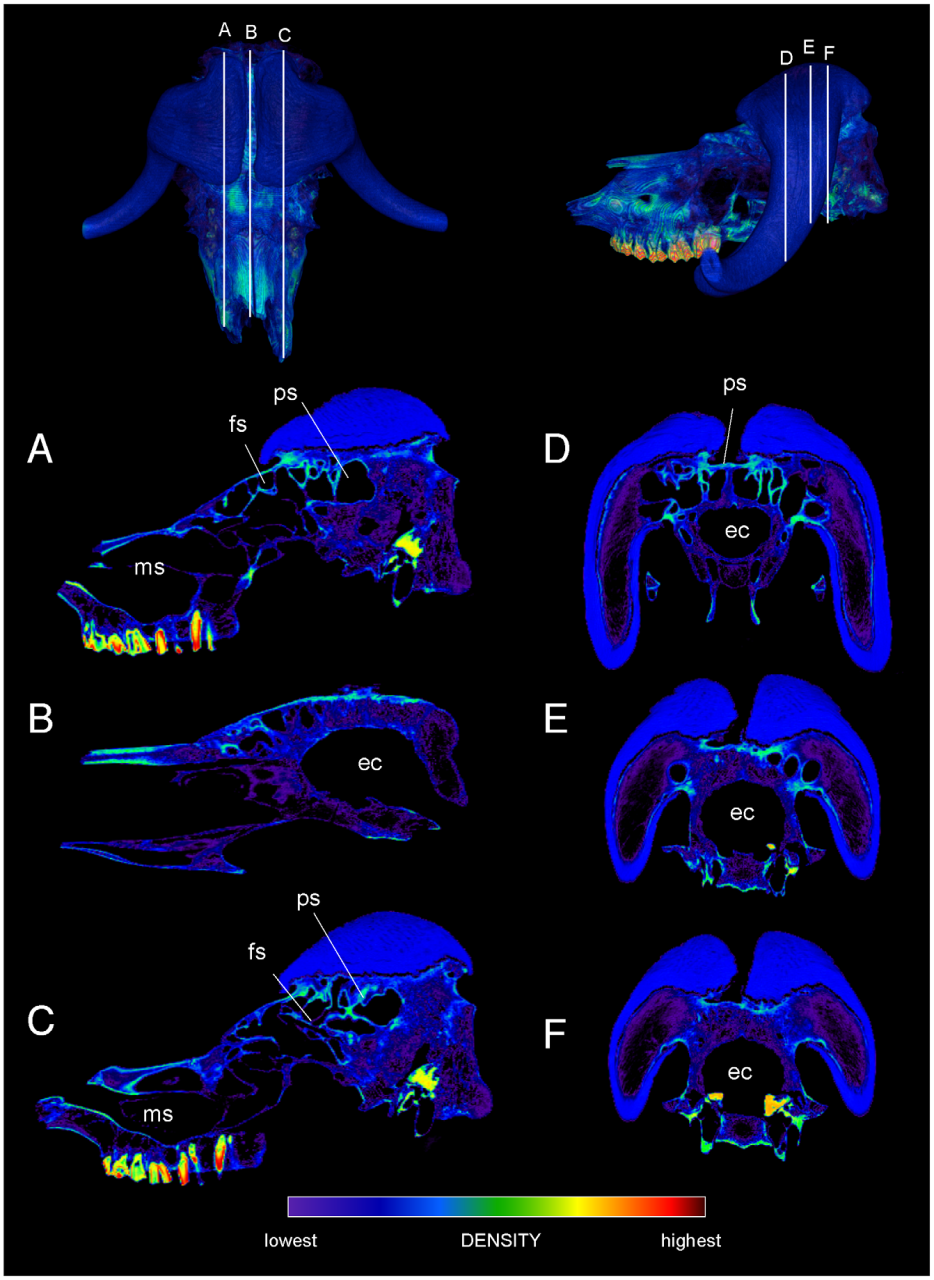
Internal densities of horn and bone in adult musk ox (*Ovibos moschatus* UCMZ M 1978.1.92). CT sections through the cranium of juvenile *Ovibos moschatus*. Dorsal and lateral CT renders show section position. A.–C. Right, mid-, and left sagittal sections show thick regions of trabeculae above the endocranial cavity, with a superficial layer of dense cortical bone over the apex of the brain (B). The most extensive cancellous bone occurs beneath the apeces of the horn sheaths, in line with the occipital condyles. A network of struts connects frontal and maxillary sinus regions (A and C). D.–F. Transverse sections from anterior to posterior show decreasing instances of struts and increasing cancellae as the horn sheaths become taller, and dense bone of the skull roof beneath the sheaths. Note the extent of trabecular bone between the sheaths and occipital condyles, in line with forces of head-butting impacts. Abbreviations: ec = endocranial cavity, fs = frontal sinus, ms = maxillary sinus, ps = parietal sinus.
